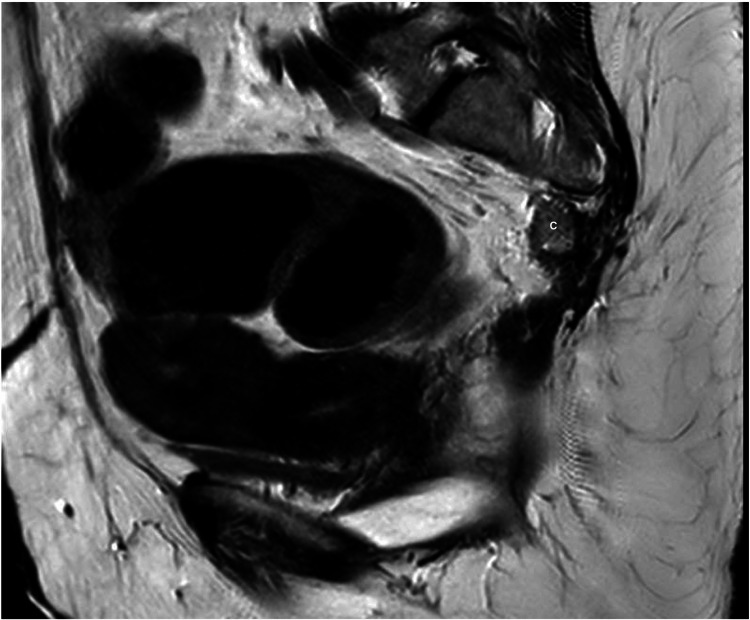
MRI pelvis T2: two-year post-resection of presacral tumor measuring 13 mm × 31 mm × 8 mm (C).
